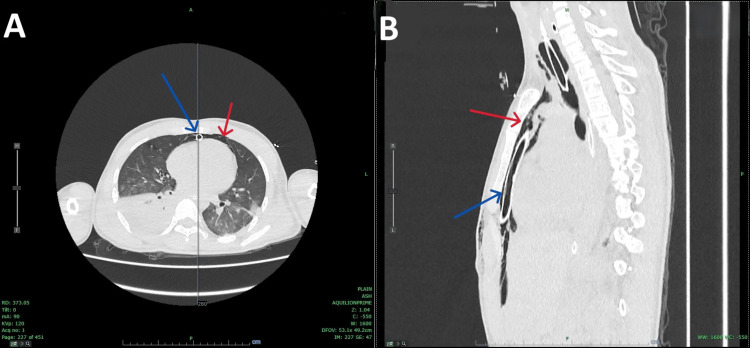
Computed tomography of the chest following insertion of the mediastinal drain (A) Axial CT view showing the mediastinal drain (blue arrow) and a significant reduction in pneumomediastinum (red arrow). (B) Sagittal CT view demonstrating the mediastinal drain (blue arrow) and markedly reduced pneumomediastinum (red arrow).
